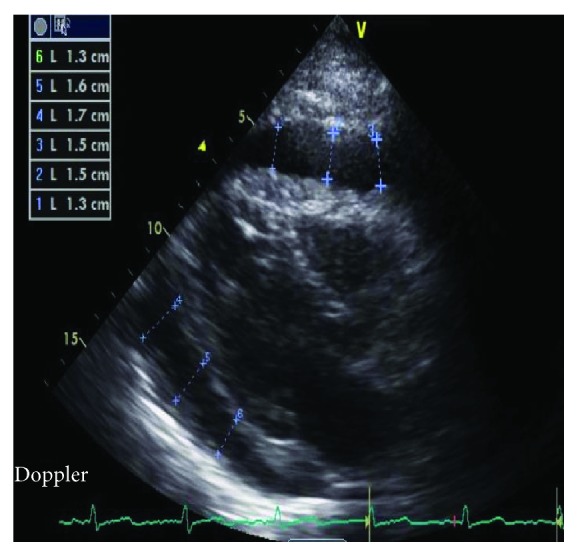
Echocardiogram demonstrating a large circumferential effusion (outlined) concerning for impending tamponade.
